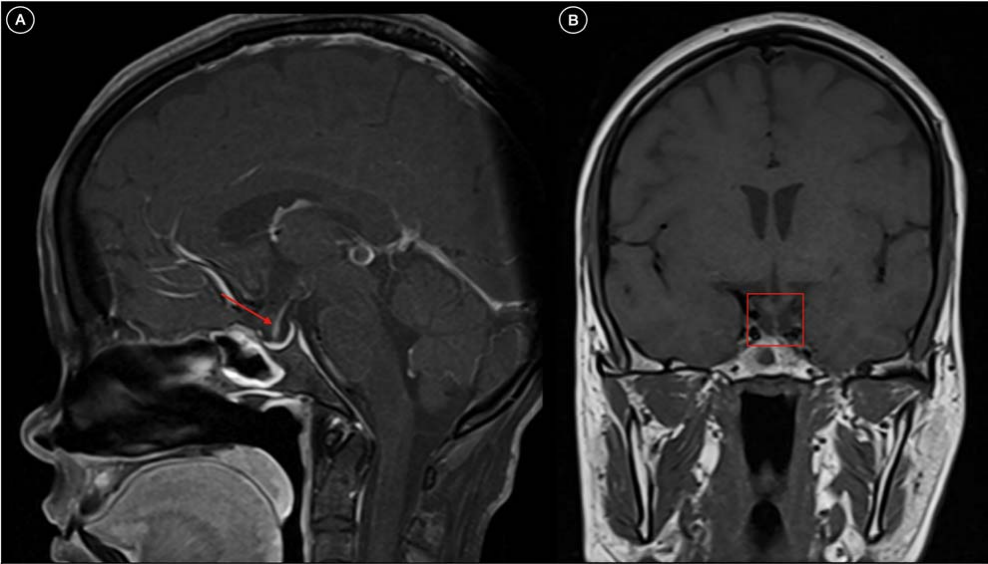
T1-weighted magnetic resonance imaging shows (A) a normal-appearing sella filled with cerebrospinal fluid with the infundibular stalk traversing to the floor of the sella (sagittal view; red arrow) and (B) an empty sella with the infundibular stalk extending to the base of the sella (coronal view; red box).
